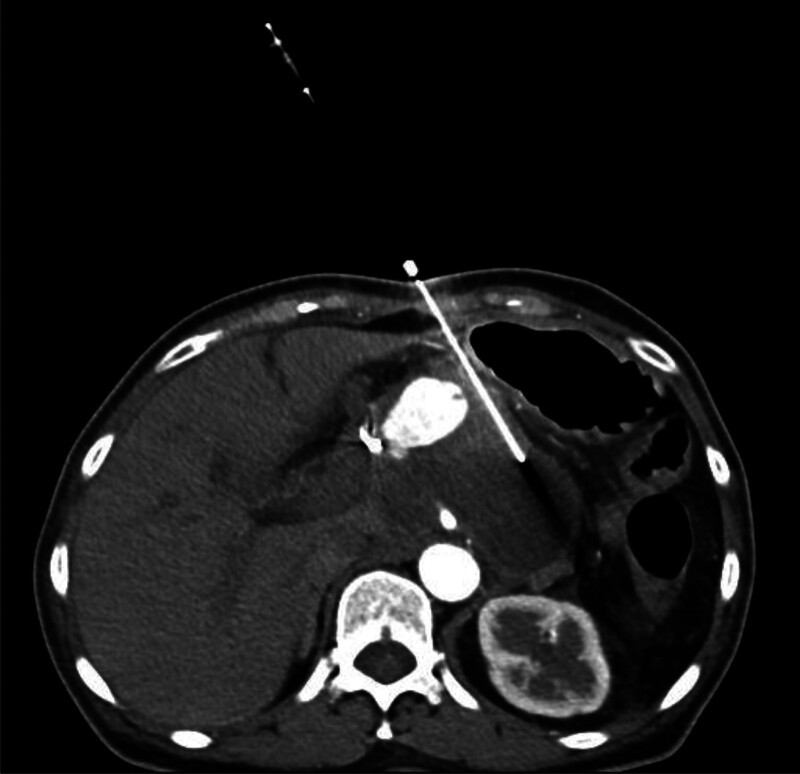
Contrast-enhanced CT scan of the abdomen during the procedure, showing a circular lesion with obvious enhancement.
